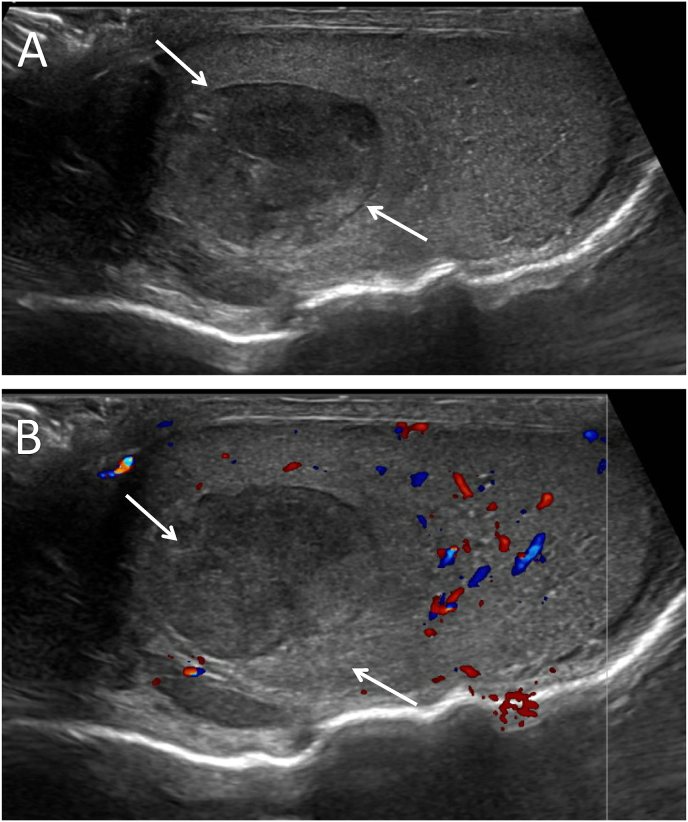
Ultrasound of right testicle showing a focal hypoechoic lesion in the right testicle (indicated by white arrows) (A), doppler ultrasound with no detectable blood flow in lesion (B).
